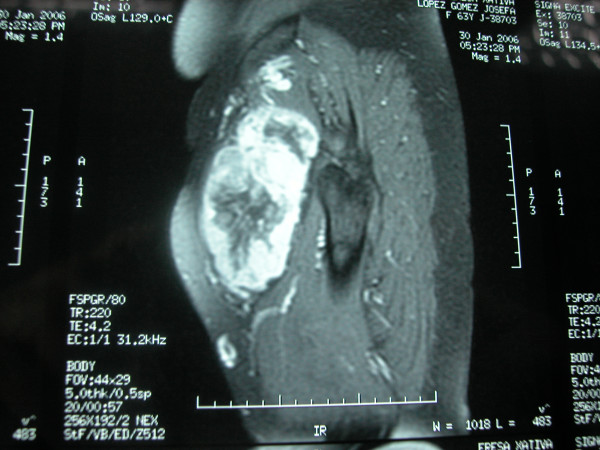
Magnetic Resonance Imaging. Tumour measuring 11 × 7 × 7 cm. in the left quadriceps.
